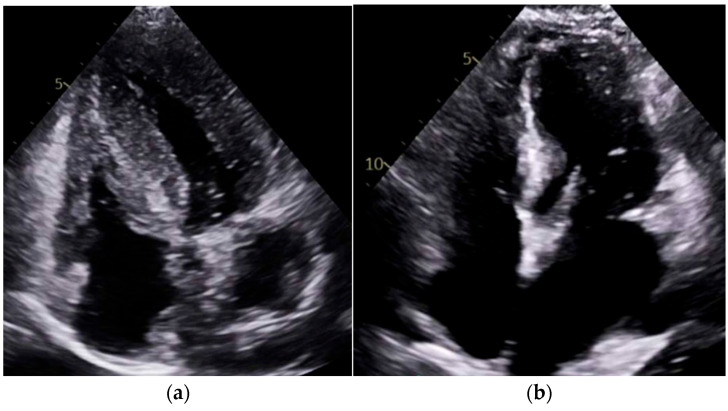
Apical four-chamber transthoracic echocardiography (TTE) comparison. (a) Cardiac amyloidosis is characterized by thickened ventricular walls, small ventricular chambers, and biatrial enlargement, consistent with restrictive cardiomyopathy. (b) Cardiac sarcoidosis showing relatively normal wall thickness, patchy wall thinning (often basal septum), and regional motion abnormalities, with atrial enlargement.
